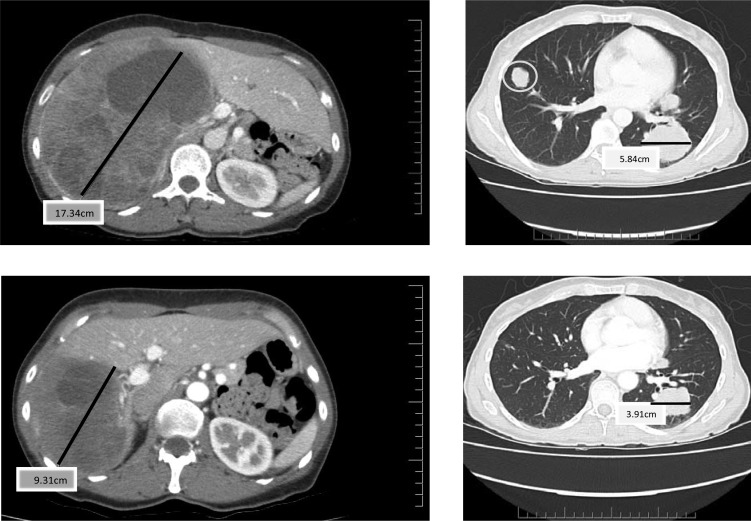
Baseline (top) and Cycle 13 (bottom) computer tomography (CT) scan (chest and abdomen) from a patient who had a partial response and a duration of 7.2 months
